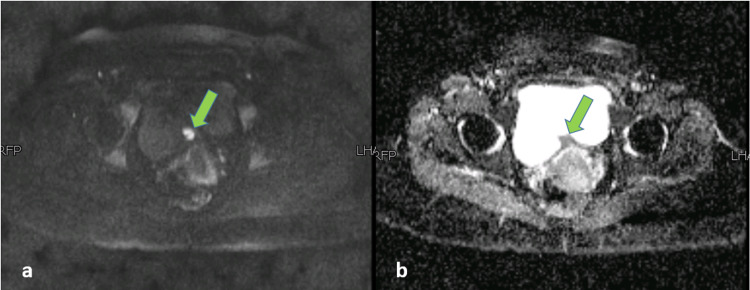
MRI pelvis - image 3 (a) Diffusion-weighted sequence and (b) apparent diffusion coefficient demonstrating the focal area of diffusion restriction within the lesion (green arrowhead) MRI: magnetic resonance imaging
